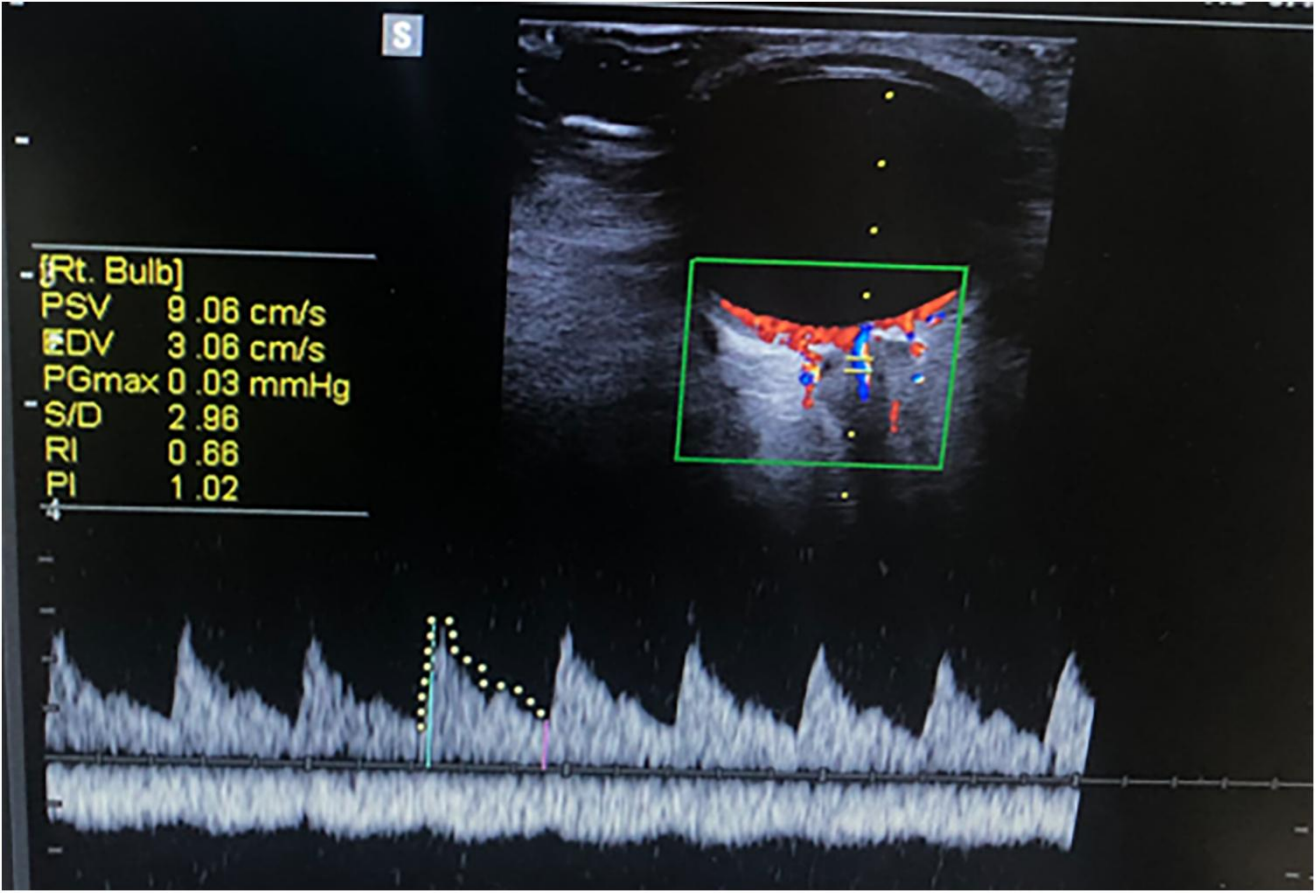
Measurement of ophthalmic artery Doppler indices.
